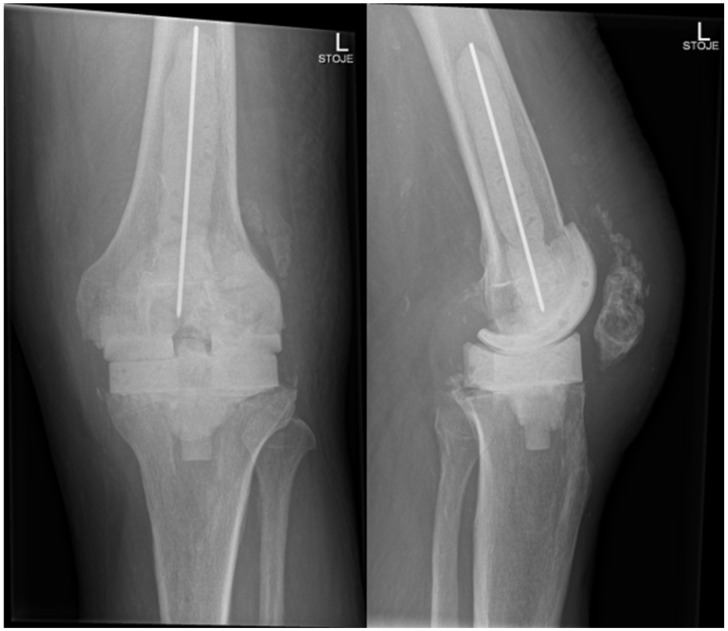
Radiography of the left knee—anteroposterior and lateral views of the knee after removal of knee endoprosthesis and insertion of an antibiotic- and antifungal-impregnated cemented spacer.
